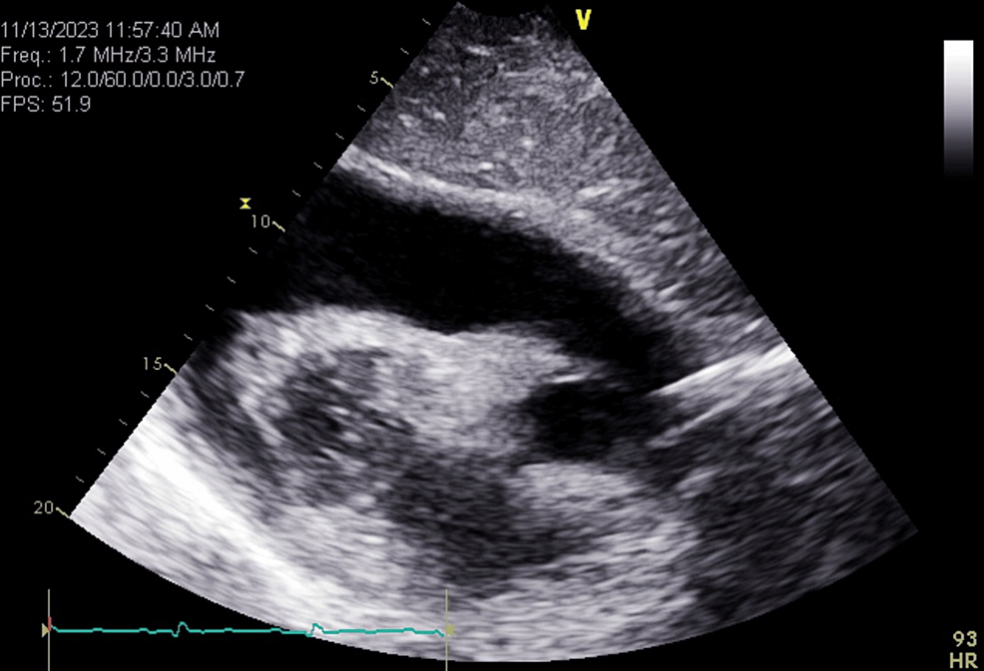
Bedside echocardiogram showing large pericardial effusion in the ED, Day 1 ED: Emergency department
